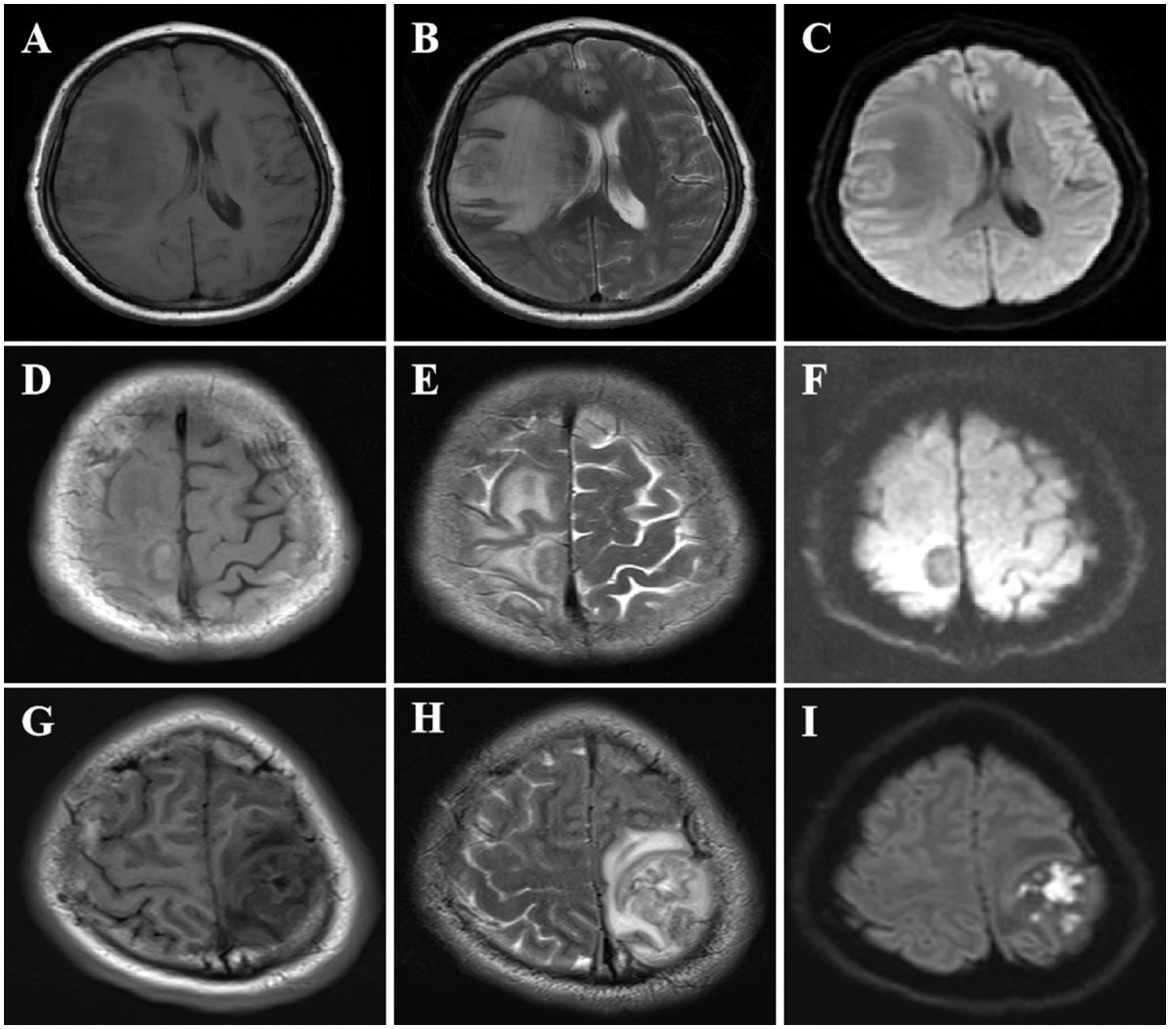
MRI imaging features of CSG. MRI imaging features of SCG. (A–C) The right frontotemporal lobe exhibited a nodular mass characterized by low signal on T1WI, high signal on T2WI, and restricted diffusion with hypointensity on DWI. (D–F) A well-defined round mass was observed in the right frontal lobe adjacent to the falx cerebri, exhibiting slightly low signal on T1WI, high signal on T2WI, and high signal surrounding the lesion on DWI. (G–I) The left parietal lobe exhibited a mass occupying lesion with slightly decreased signal intensity on T1WI, iso-high signal intensity on T2WI, and high signal intensity on DWI, iso-high signal intensity surrounding the lesion.
